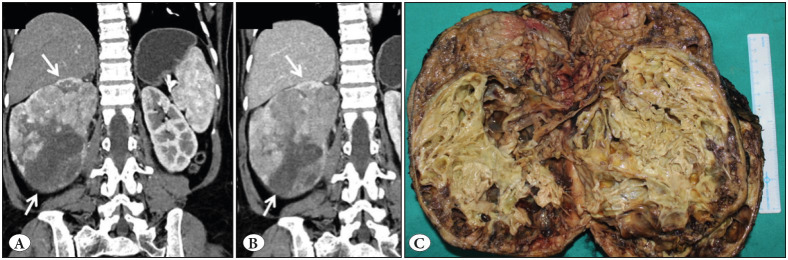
A) Coronal CT image in corticomedullary phase shows large malignant mass in the right kidney (arrows) with heterogeneous moderate enhancement and a large area of necrosis. B) Nephrographic phase scan showing same pattern of moderate enhancement with no washout (arrows). C) Growth is solid cystic, predominantly yellowish and necrotic in the inferior and middle poles and fleshy tan brown in the upper pole.
